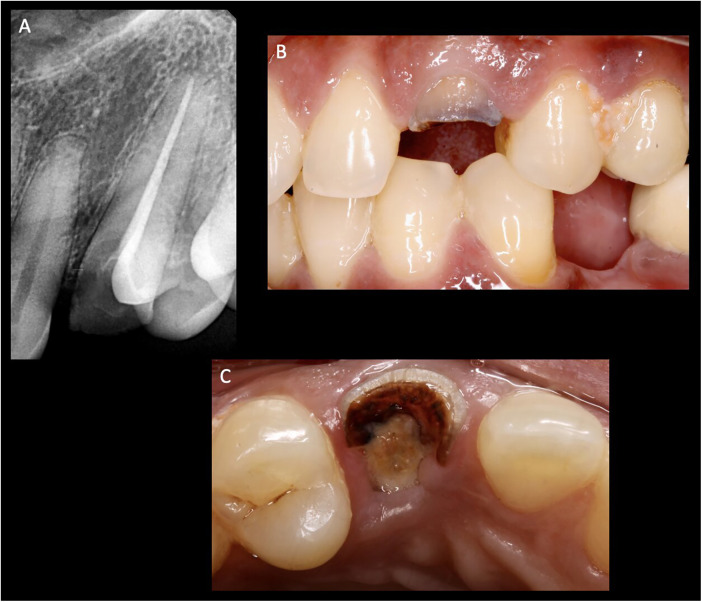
Preoperative assessment of tooth 23. (A) Preoperative intraoral periapical radiograph showing a previously root canal–treated tooth with satisfactory obturation and absence of periapical pathology. (B) Preoperative clinical view demonstrating extensive coronal destruction of tooth 23. (C) Occlusal view showing the structurally compromised tooth with palatal involvement.
